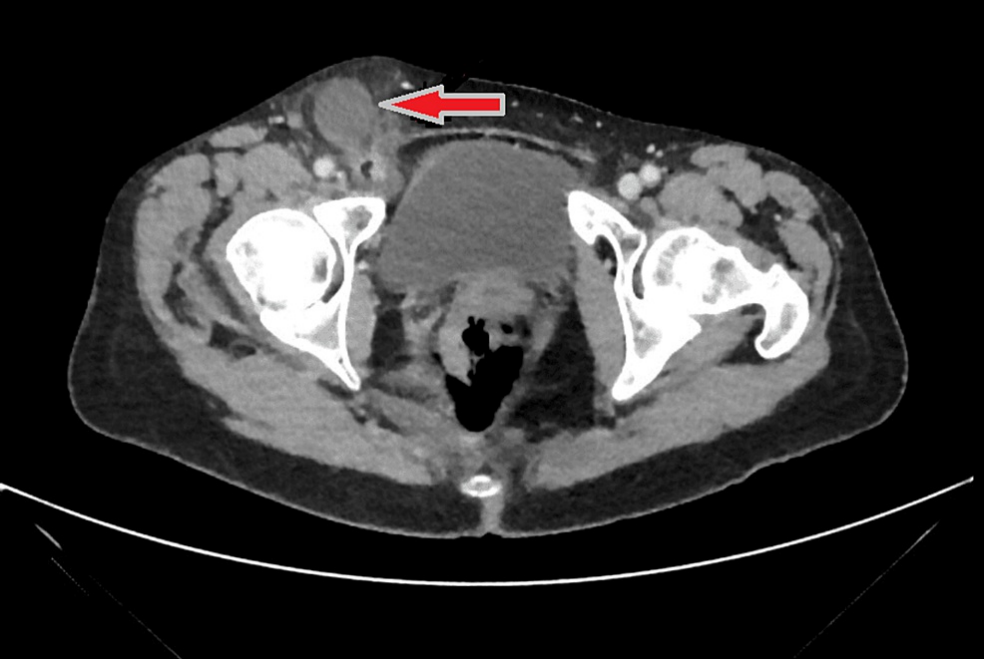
CT abdomen pelvis indicated possible perforated acute appendicitis with 3.2x4.3 cm fluid collection (appendiceal tip abscess) within a femoral hernia CT, computed tomography
